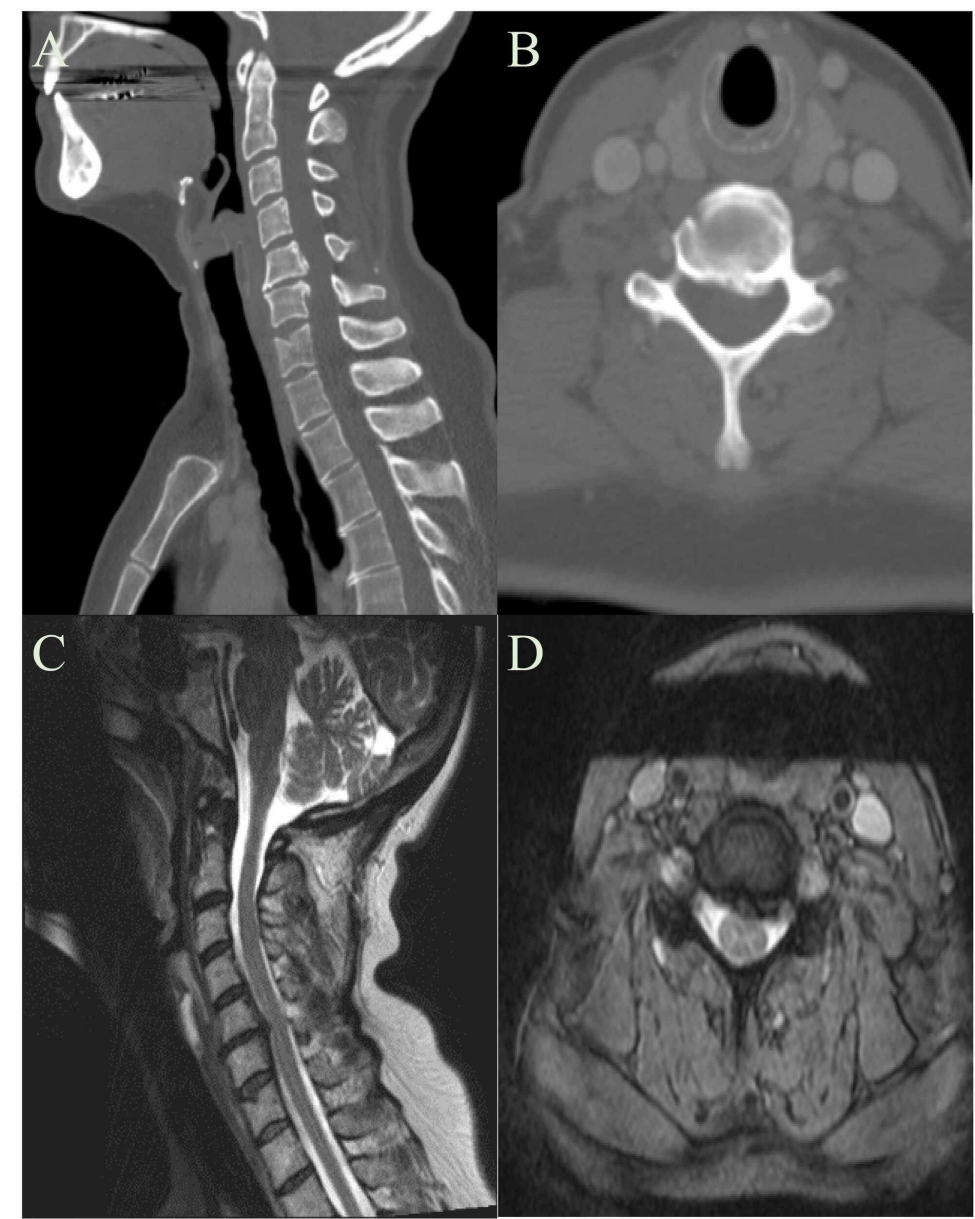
Patient 2 CT and MRI of the Neck A: Patient 2 CT Neck Sagittal View B: Patient 2 CT Neck Axial View C: Patient 2 MRI Neck Sagittal View D: Patient 2 MRI Neck Axial View A disc osteophyte complex with narrowing of the spinal canal is seen at C5-6 (Cervical Vertebrae 5-6).
